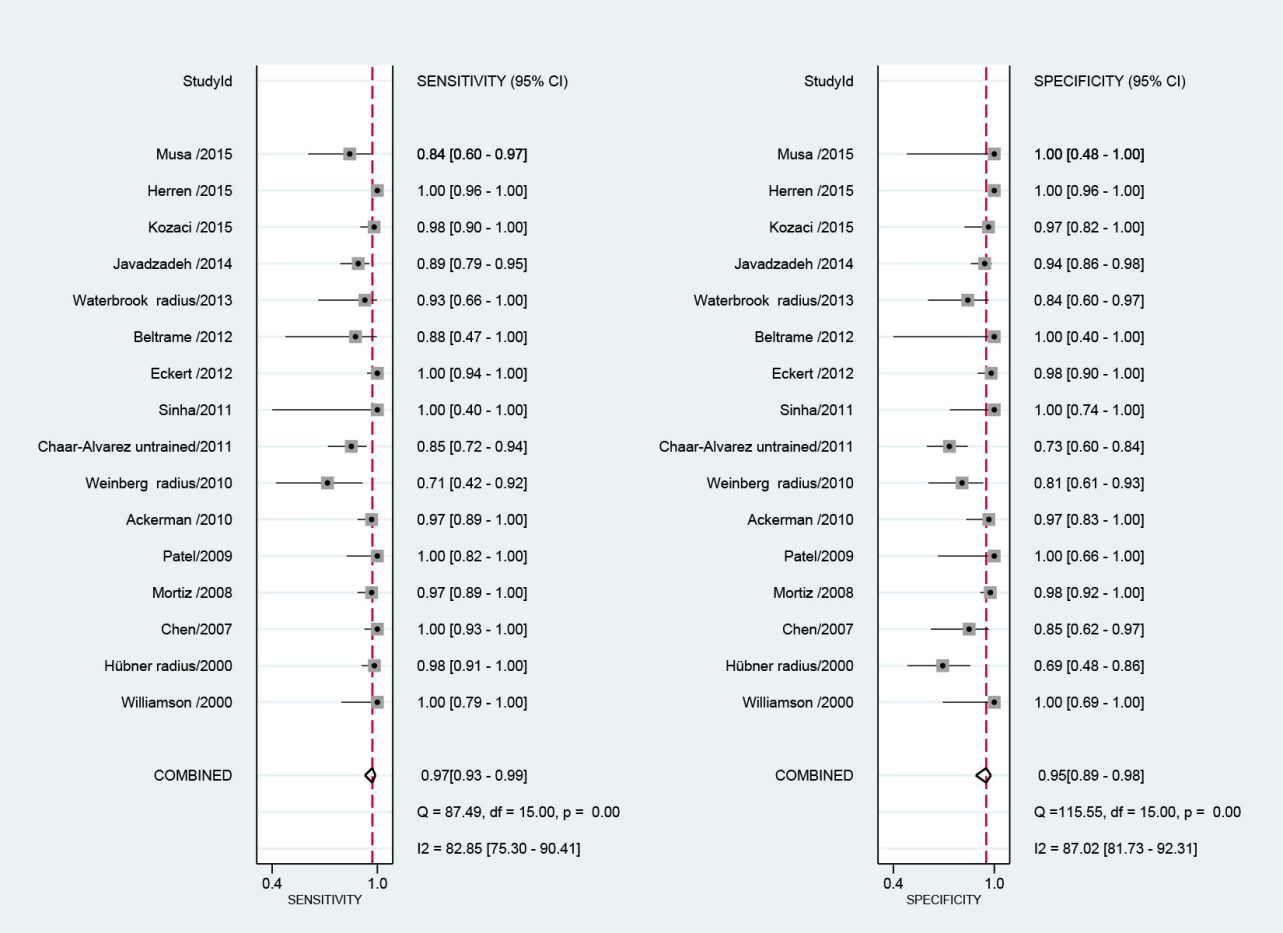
Coupled Forrest plots sensitivity and specificity for ultrasound compared to conventional x-ray, for the diagnosis of distal forearm fracture.
